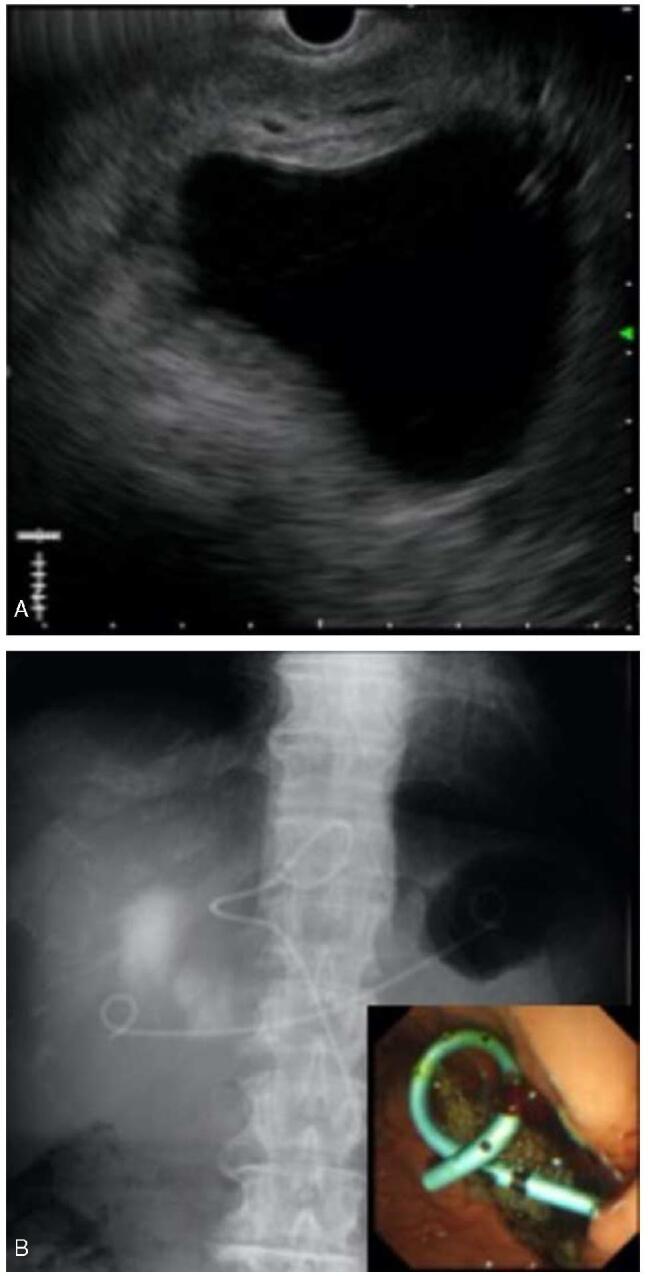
Figure 5. Endoscopic ultrasound-guided drainage was performed for the liver cyst with biliary communication. A) Endoscopic ultrasound showing a liver cyst. B) A 7 Fr × 15 cm double pigtail plastic stent was placed in the liver cyst.
